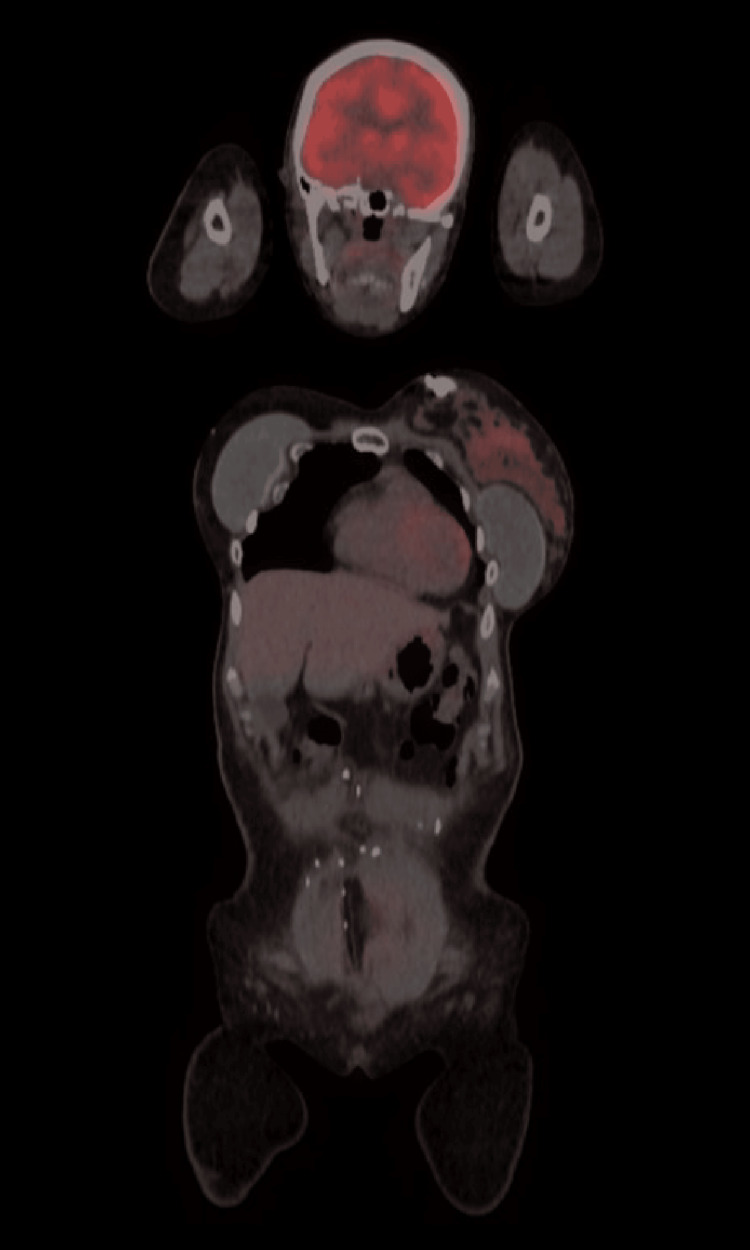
Sagittal PET-CT view of the case status postpartum with no evidence of disease and only physiological uptake findings in the left breast with no metastasis. PET-CT, positron emission tomography-computed tomography
